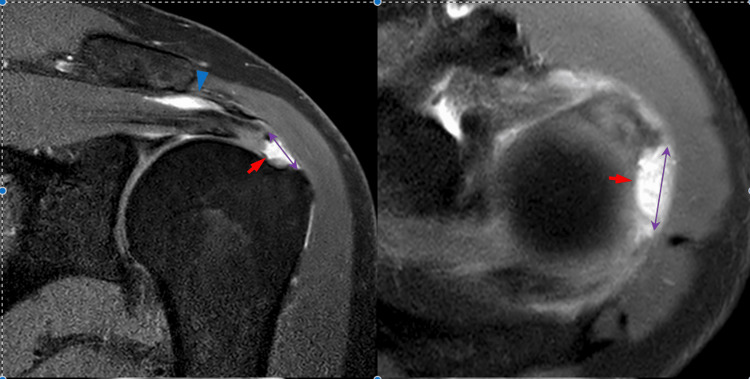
Full-thickness rotator cuff supraspinatus tear on MRI Left: Coronal view of shoulder joint on MRI. The blue arrowhead indicates a full-thickness/transmural tear of the distal supraspinatus tendon (red arrow) with increased fluid in the subacromial/subdeltoid bursa. Right: Shoulder joint MRI axial view T1. The red arrowhead shows mild long-head biceps tendinosis. The purple arrow indicates the measurement of the mediolateral and anteroposterior diameter of the supraspinatus muscle. Image source: Ref. [10].
